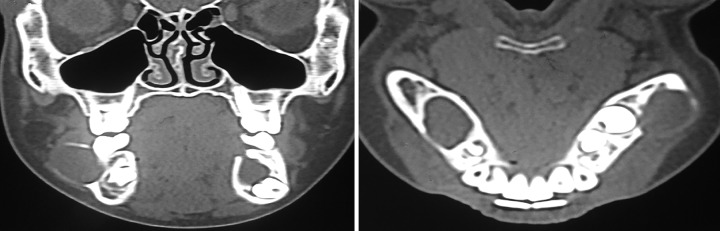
Computed tomography mandible showing bilateral OKCs
